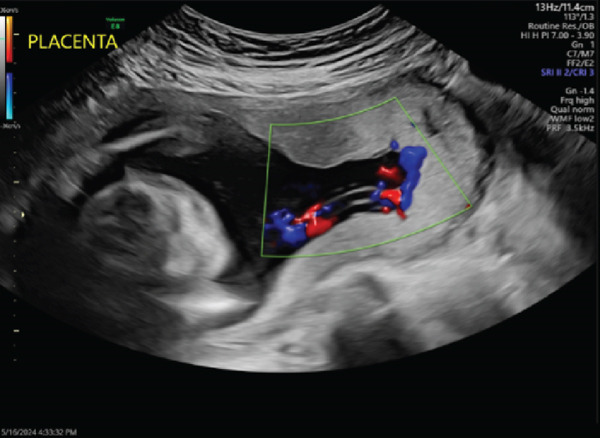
Ultrasound findings of the left lateral placenta without evidence of PAS at 36 weeks′ gestation.
